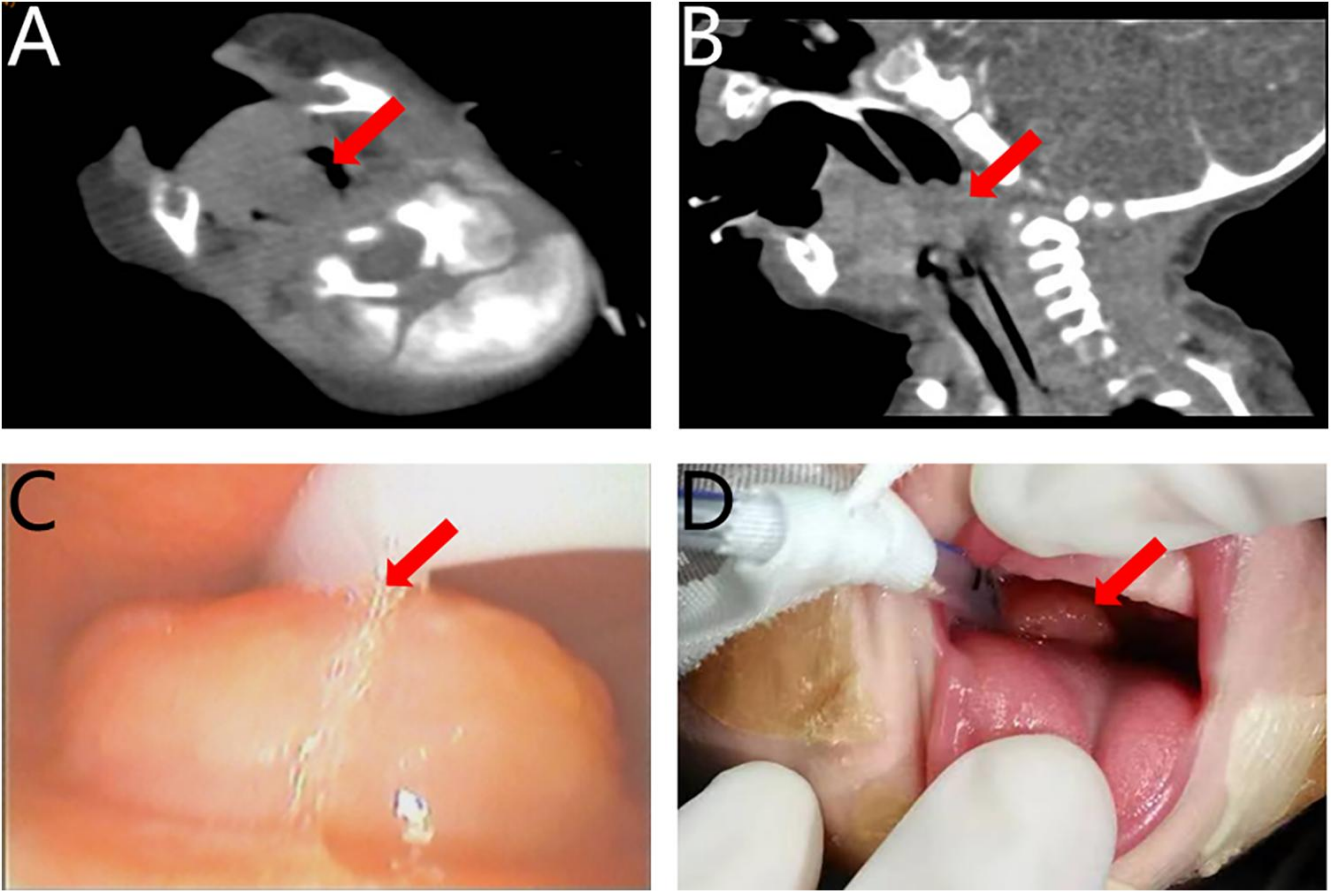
(A,B) CT showing the tumor arising from the base of tongue (red arrow). (C) Fiberoptic bronchoscopy revealing the tumor at the base of the tongue (red arrow). (D) Preoperative intraoral view showing a mass at the tongue base (red arrow).
